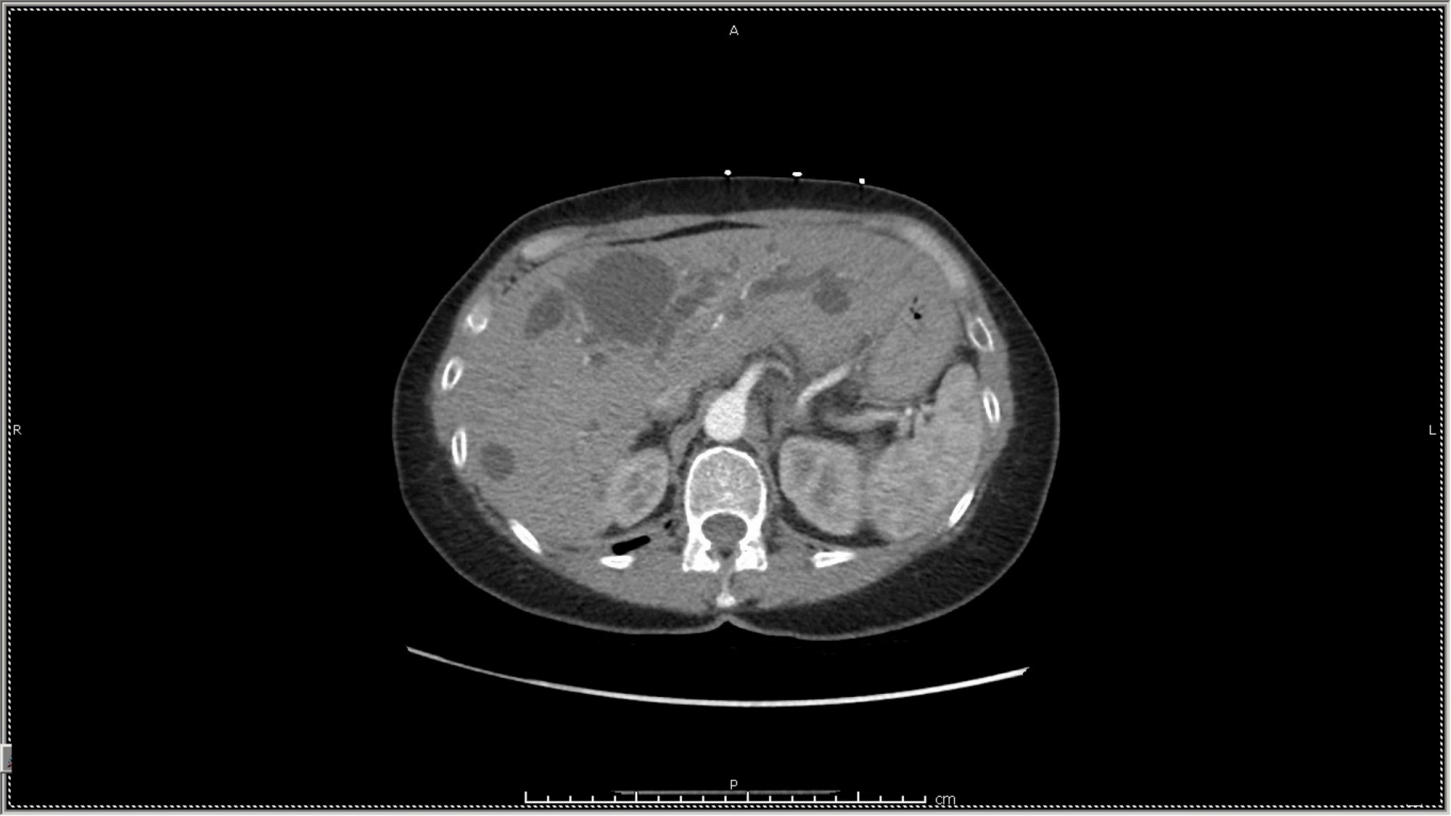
Liver Computed Tomography Scan Multiple cysts visible in the liver.
